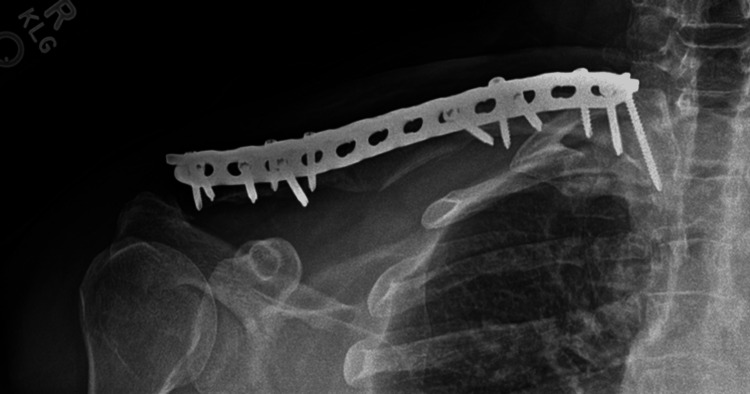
Six-month postoperative radiograph showing the integration of the bone graft and fracture union.
